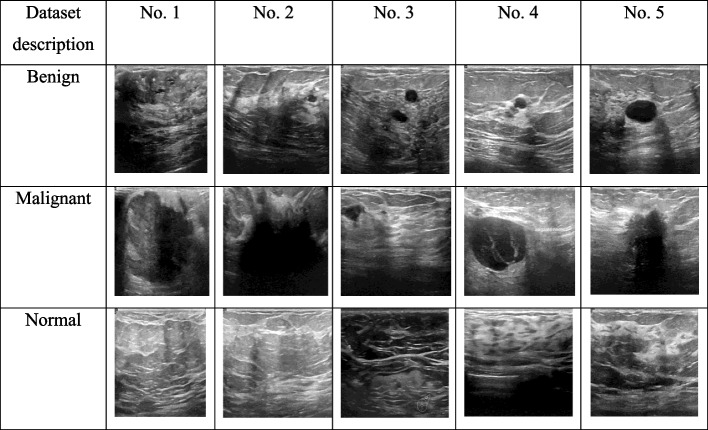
Gathered ultrasound images from online resources
